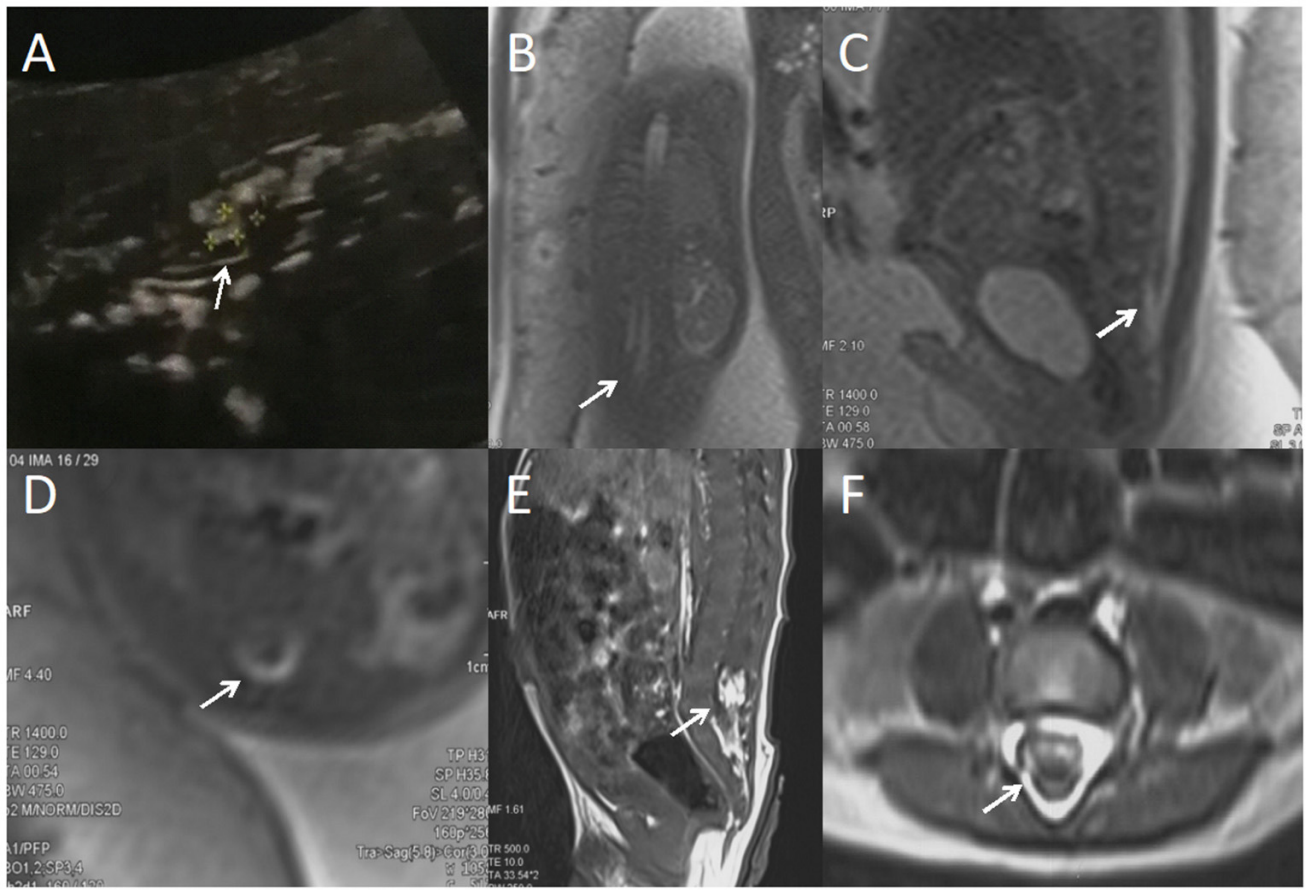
A fetus with intraspinal lipoma. (A) the prenatal ultrasound examination at 24 weeks of gestation showed the presence of low conus medullosus in the fetus, hyperechoic mass shadow in the spinal canal, and broken spinal continuity. Thus, spina bifida and intraspinal lipoma were considered. (B) Fetal magnetic resonance imaging (MRI) examination at 24 + 2 weeks of gestation showed a coronal view of the low conus medullosus of the fetus, with enlarged end of the conus medullosus and tight adhesion to the posterior edge of the spinal canal. (C) Sagittal view showed the low conus of fetal myeloma cord. (D) In the axial position, normal subarachnoid space in the spinal canal disappeared, with lipoma and spinal nerves mixed. (E) T1 sagittal MRI of the lumbosacral vertebrae 10 days after birth suggested intraspinal lipoma. (F) Axial MRI of the lumbosacral vertebrae showed uneven internal signal of lipoma, which was considered to be a hybrid lipoma with the spinal nerves.
